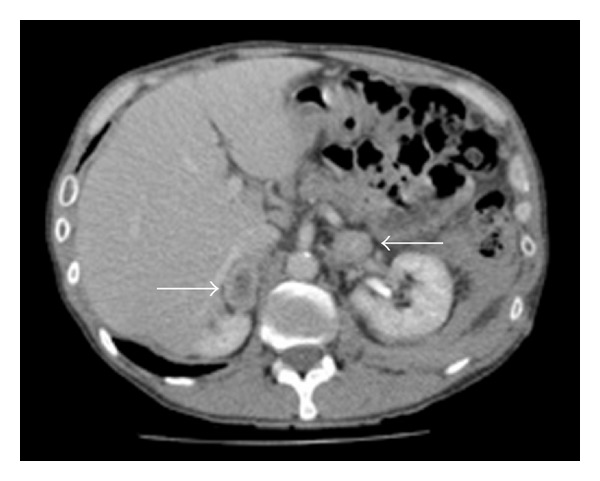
Axial CT image at the level of the adrenal glands demonstrating bilateral adrenal masses (arrows).
